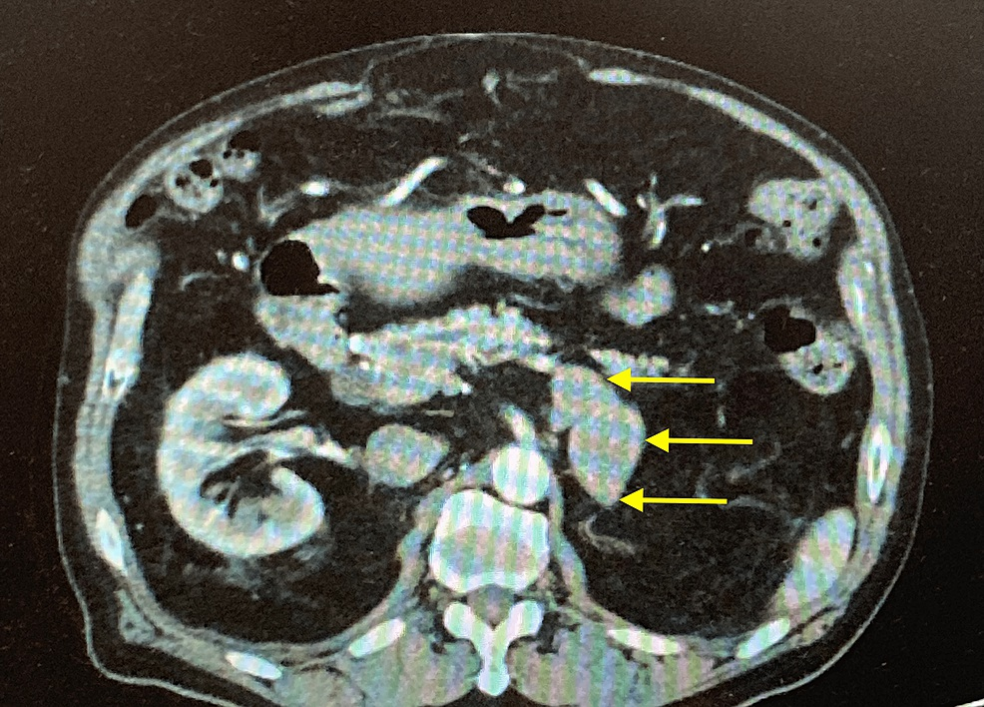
Contrast-enhanced CT of the abdomen Yellow arrows indicate the adrenal tumor measuring 58 mm x 31 mm x 55 mm.
